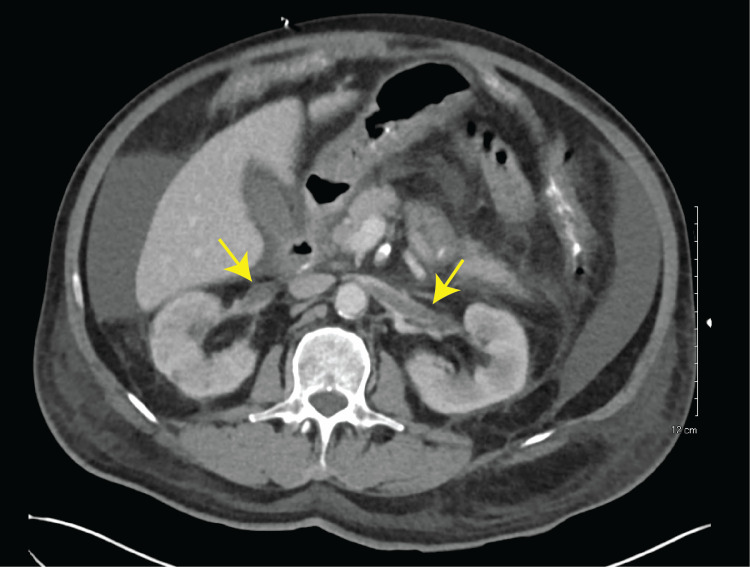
Abdominal CT scan with contrast revealed bilateral renal vein thrombosis and ascites Abdominal CT scan with contrast showed bilateral renal vein thrombosis. Yellow arrows indicate renal vein thrombosis.
